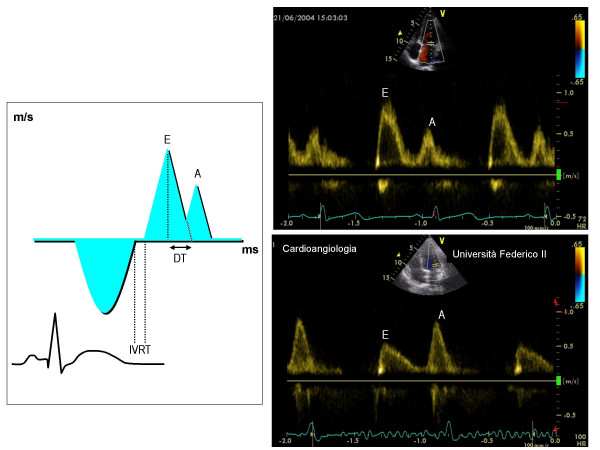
In the left screen, methodological outline for the measurement of Doppler transmitral indexes of diastolic function. In the right screen, normal diastolic pattern (upper part) and pattern of abnormal relaxation (lower part). A = atrial velocity (m/s), DT = deceleration time of E velocity (ms), E = early diastolic velocity (cm/s), IVRT = isovolumic relaxation time (ms)
